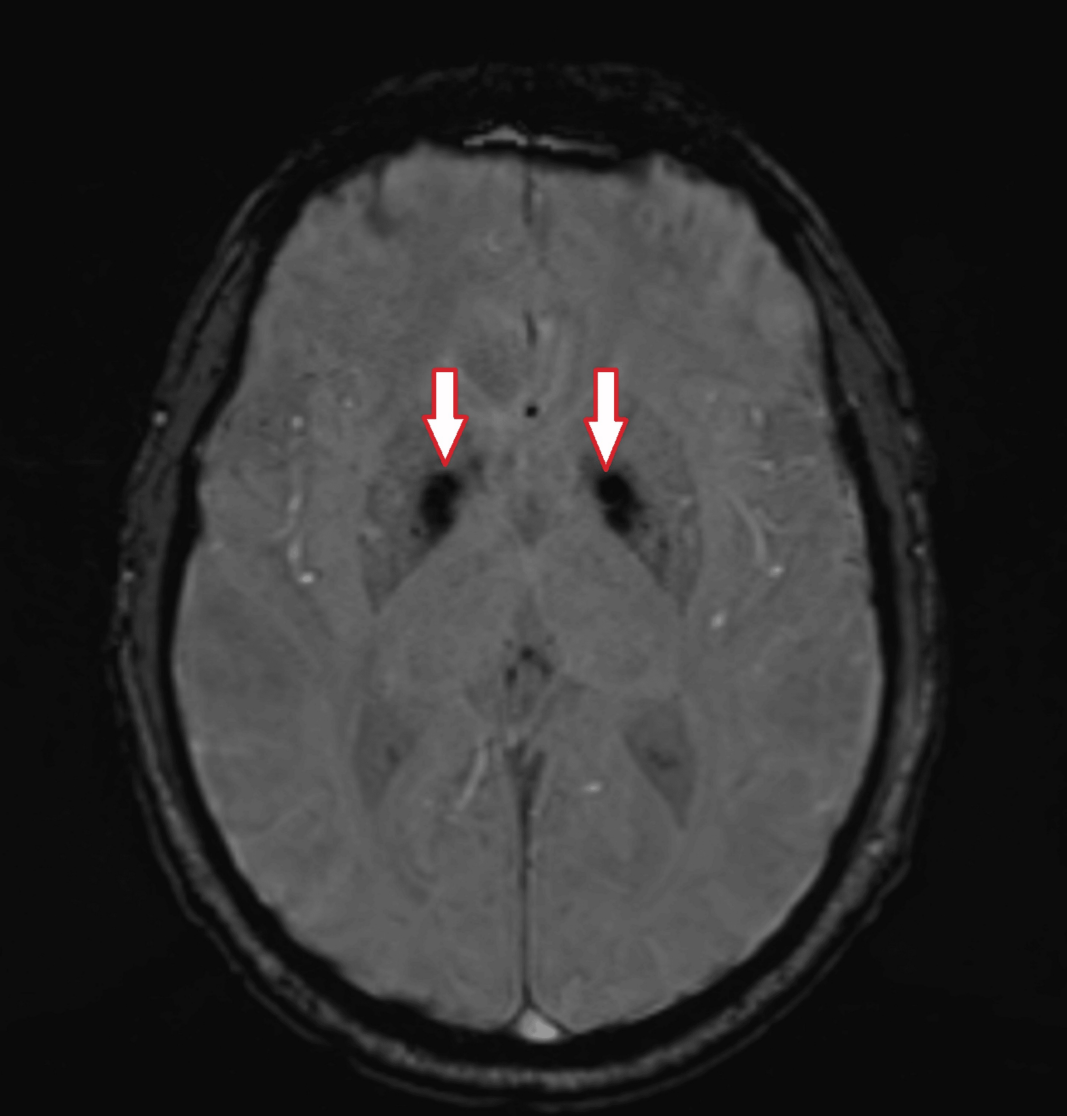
Axial susceptibility-weighted imaging (SWI) MRI of the brain demonstrating marked hypointensities in the bilateral basal ganglia, consistent with mineral (calcium) deposition (arrows).
